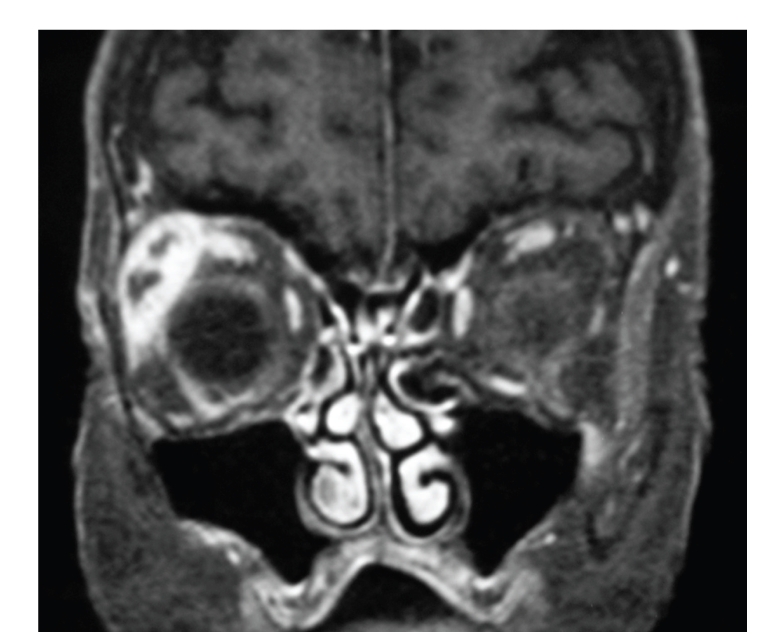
T1 weighted contrast enhanced MRI scan coronal sections through the orbits showing contrast enhancing lacrimal gland lesions with central hypodense areas.
